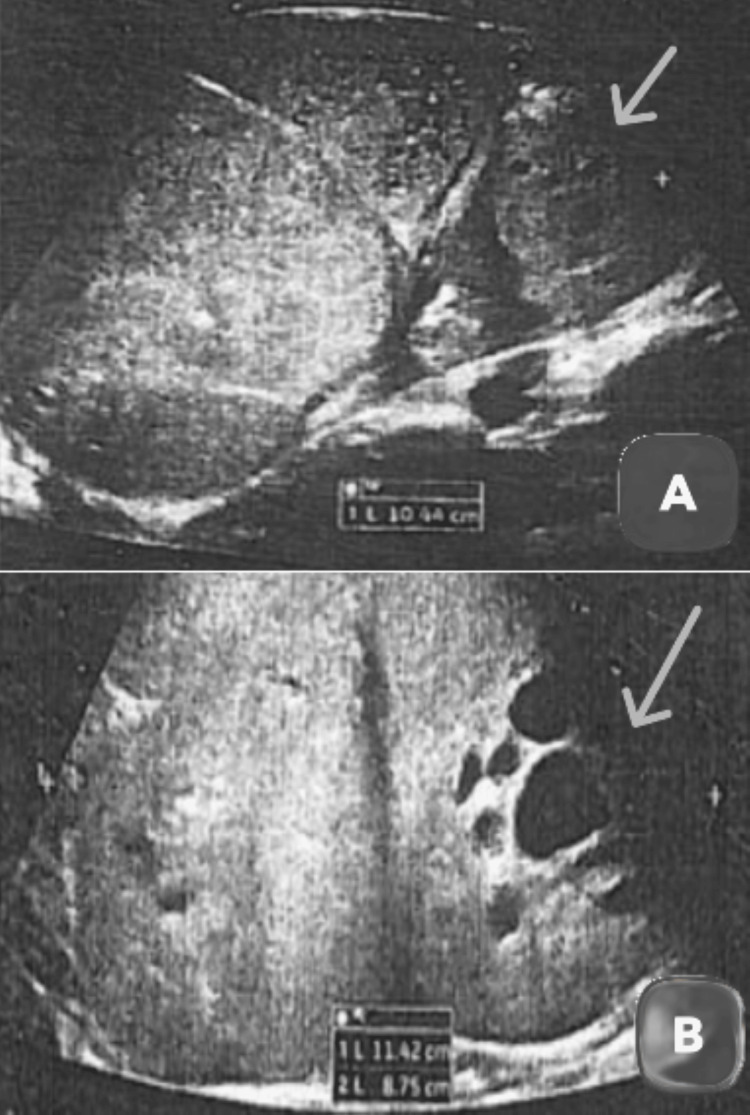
Initial abdominal ultrasound of the patient A: Enlarged left kidney with identification of multiple well-defined, rounded, heterogeneous isoechoic masses containing cystic areas, the largest measuring 104 × 78 mm in its greatest dimensions, as seen in the upper image. B: A large mass centered in the right hypochondrium and right flank, well-defined with lobulated contours, of heterogeneous echostructure, containing cystic areas, measuring 114 × 87.5 mm, as seen in the lower image.
